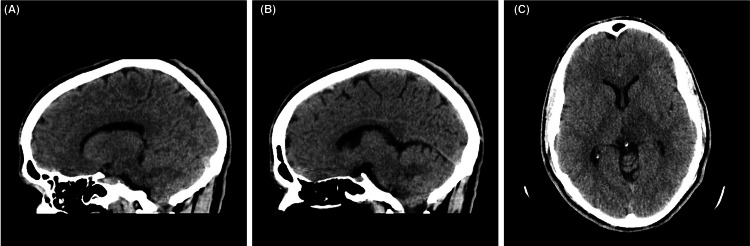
CT scan images (A) and (B) demonstrate a sagittal axis view, while image (C) demonstrates an axial axis view. This figure demonstrates the presence of a left thalamic lesion with involvement of the internal capsule’s posterior limb.
